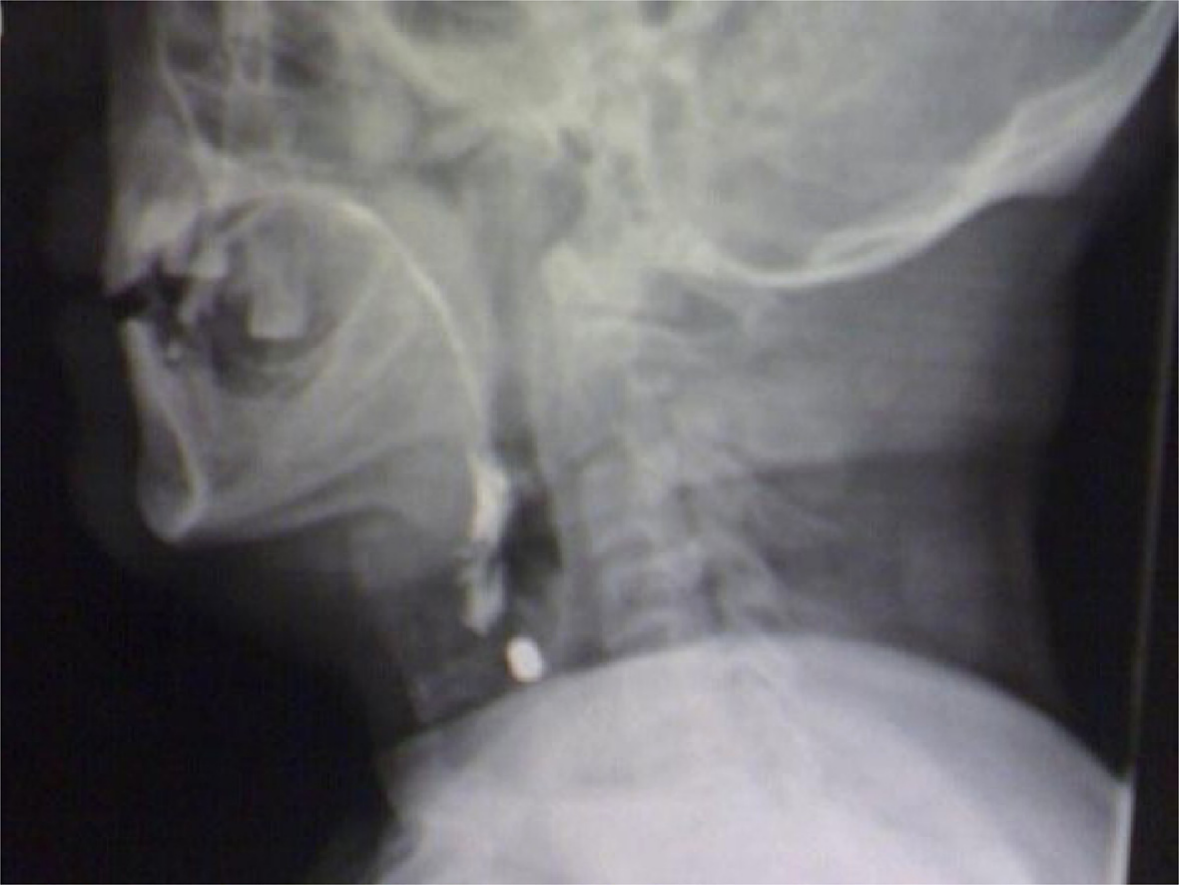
Plain cervical X-ray (Lateral views) of the patient. Screening X-rays showed the location of the pellet.
